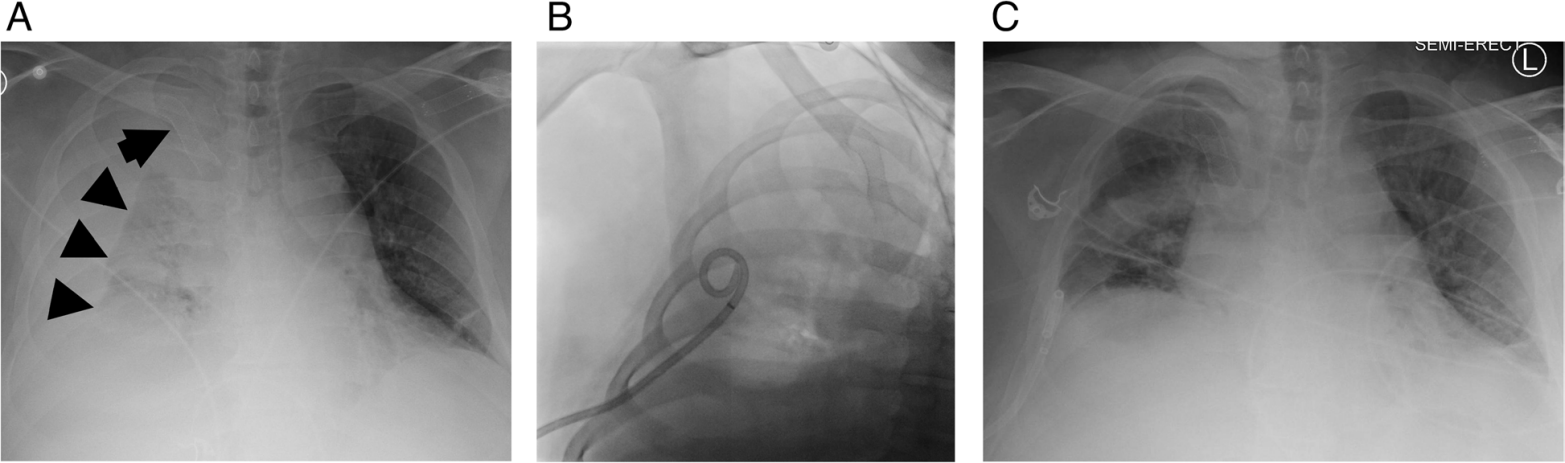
Complication following successful RF-wire enabled recanalization and stenting of a right brachiocephalic occlusion. A Portable chest radiograph obtained immediately following procedure showing stent graft in good position (arrow) but the presence of a large right hemothorax (arrowheads). B Locking loop 14 French chest tube was placed in the IR suite, yielding 800 mL of blood. C Chest radiograph obtained 48 h later showing resolution of hemothorax. The chest tube was subsequently capped and then removed
